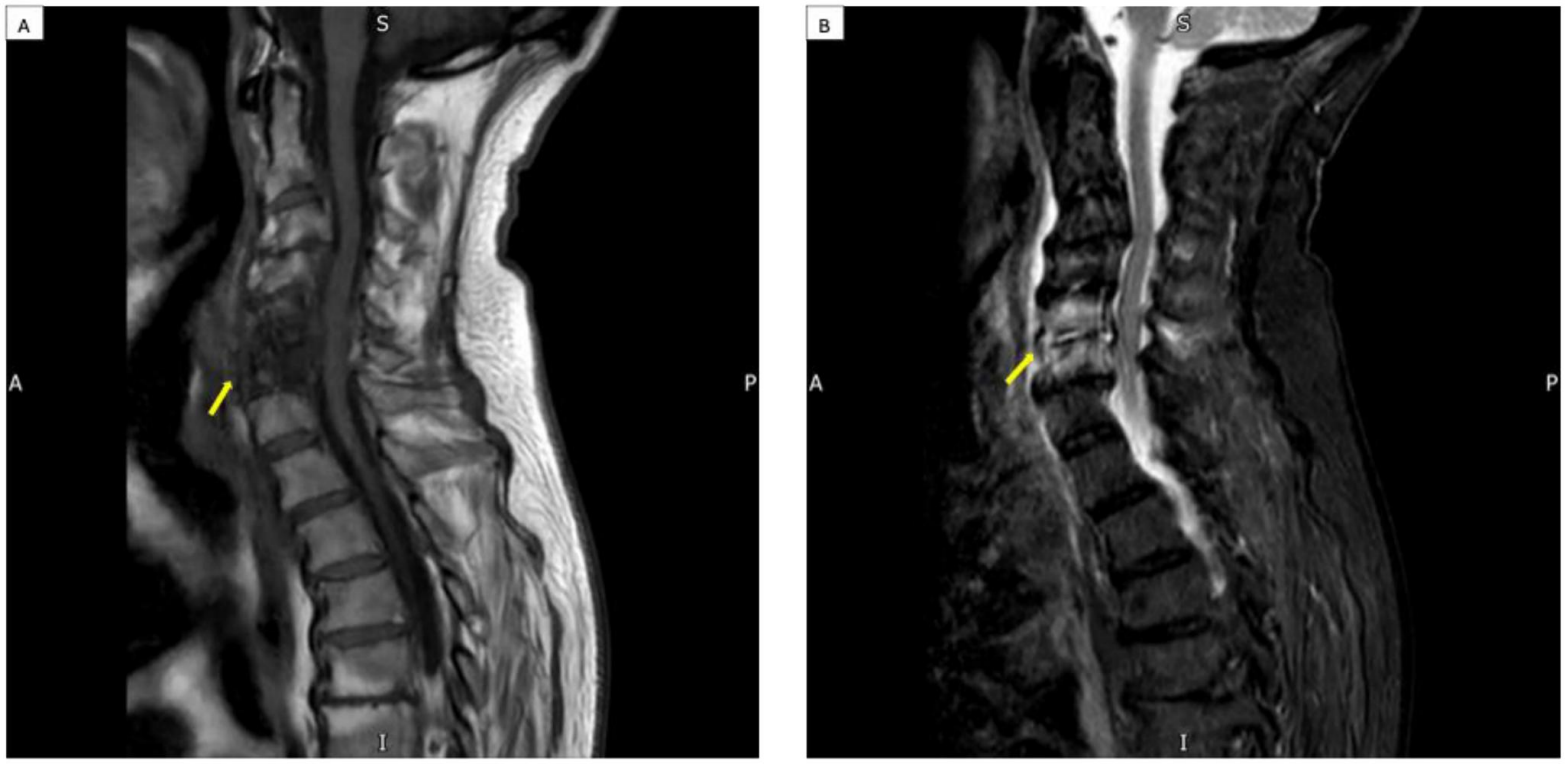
MRI cervical spine T1-weighted image (A) and sagittal STIR (B) showing bone marrow oedema and end-plate changes at C5/6 with a small abscess also developing at this level due to a second level of spondylodiscitis (axial sequences not shown).
